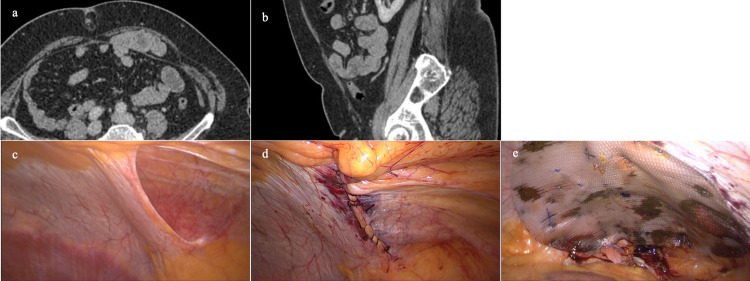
Axial (a) and sagittal (b) computed tomography with intravenous contrast depicting a left-side linea arcuate hernia containing the small bowel. Laparoscopic photographs of the internal defect (c) with primary suture repair (d) and reinforcing prosthetic mesh with hydrogel barrier (e).
